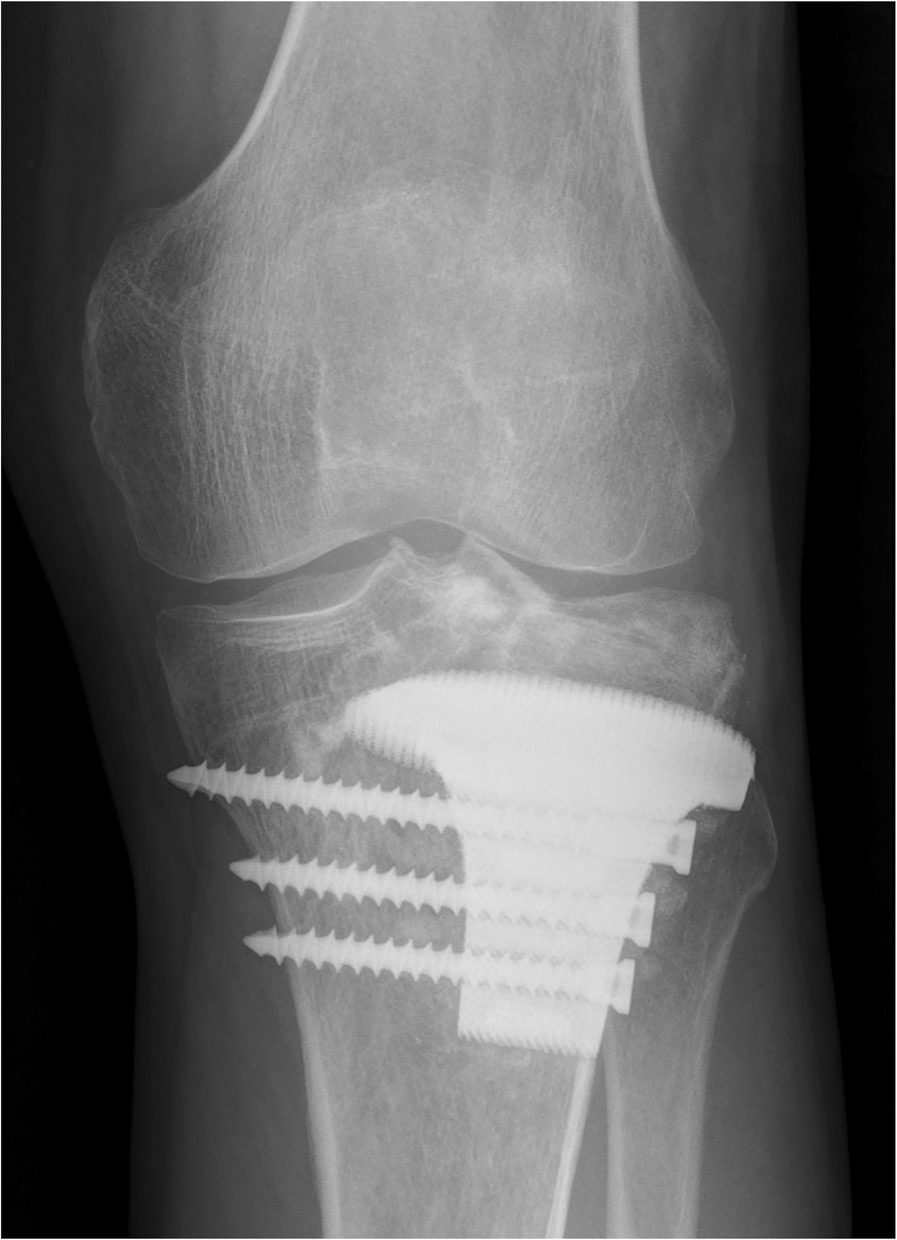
Anteroposterior radiograph of left tibia 29 months after 3D-printed implant reconstruction
